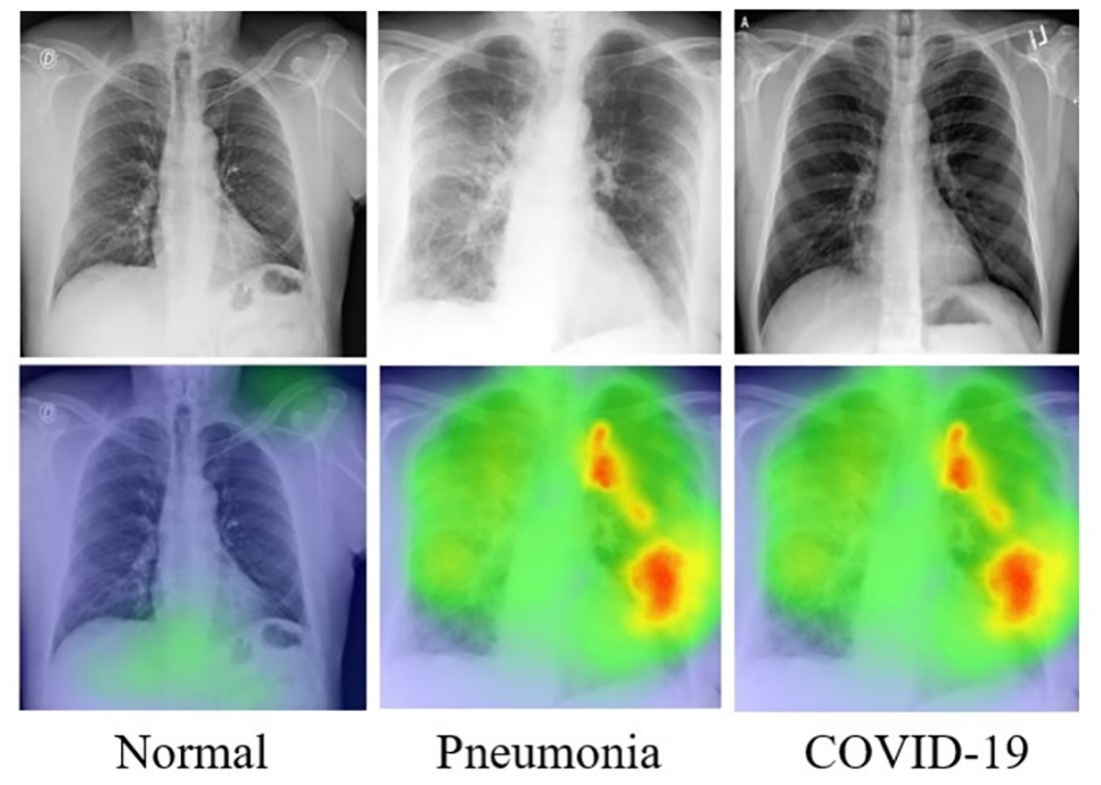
Visualization of chest X-ray images using Grad-CAM on Dense MobileNetV3 model.
